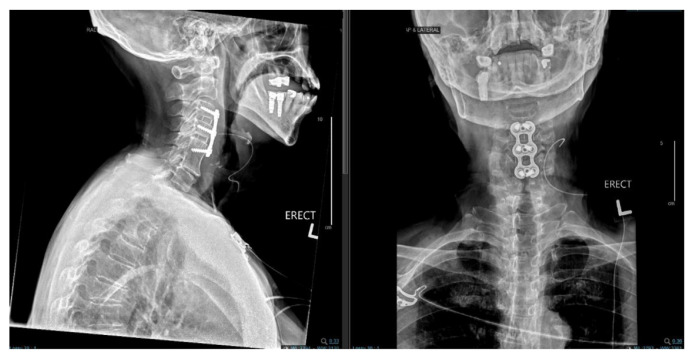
Immediate post op X-rays after C3–C5 ACDF demonstrating satisfactory placement of the implants.
